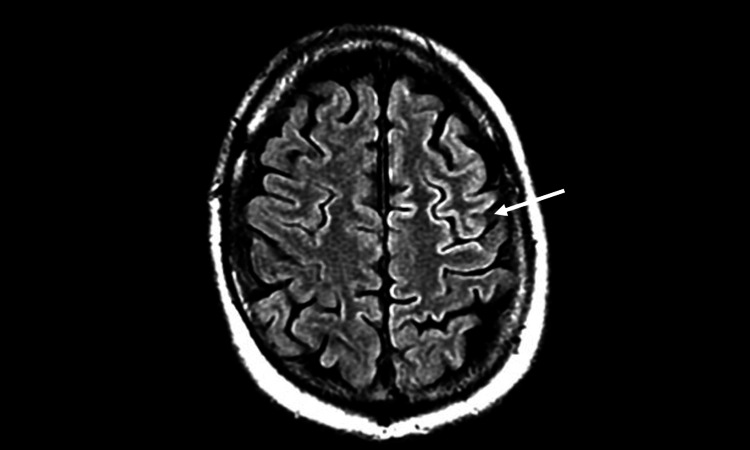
Increased T2 FLAIR signal changes in the frontal lobe FLAIR: fluid-attenuated inversion recovery
